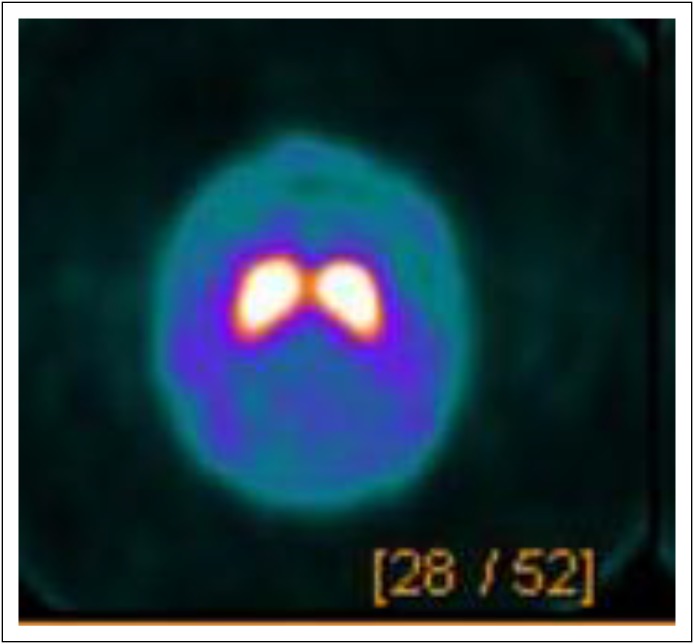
Brain SPECT DaTscan. Normal dopamine transporter uptake in the bilateral
